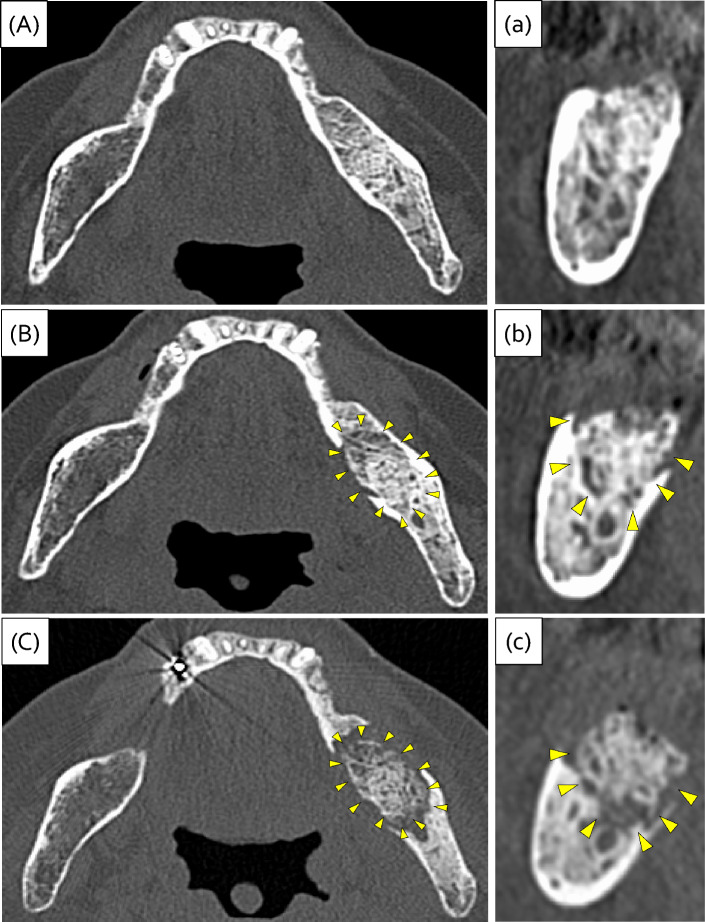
Temporal CT images of a patient with left mandibular MRONJ. A First axial CT image. Entire osteosclerosis is visible in the left mandible. a First coronal CT image. * mandibular canal. B Second axial CT image. A new area of mixed osteosclerosis and osteolysis 3 months after image (A). The visible line dividing this area from the osteosclerotic lesion is “MRONJ demarcation line” (yellow arrowheads). b Second coronal CT image. MRONJ demarcation line is the line between the lesion with incomplete sequestration and the osteosclerotic mandible. C Third axial CT image. The lesion consistent with MRONJ demarcation line is completely sequestrated from the left mandible 8 months after image (A). c Third coronal CT image. The boundary between the necrotic lesion and the osteosclerotic area is clear. *CT* computed tomography, *MRONJ* medication-related osteonecrosis of the jaw
